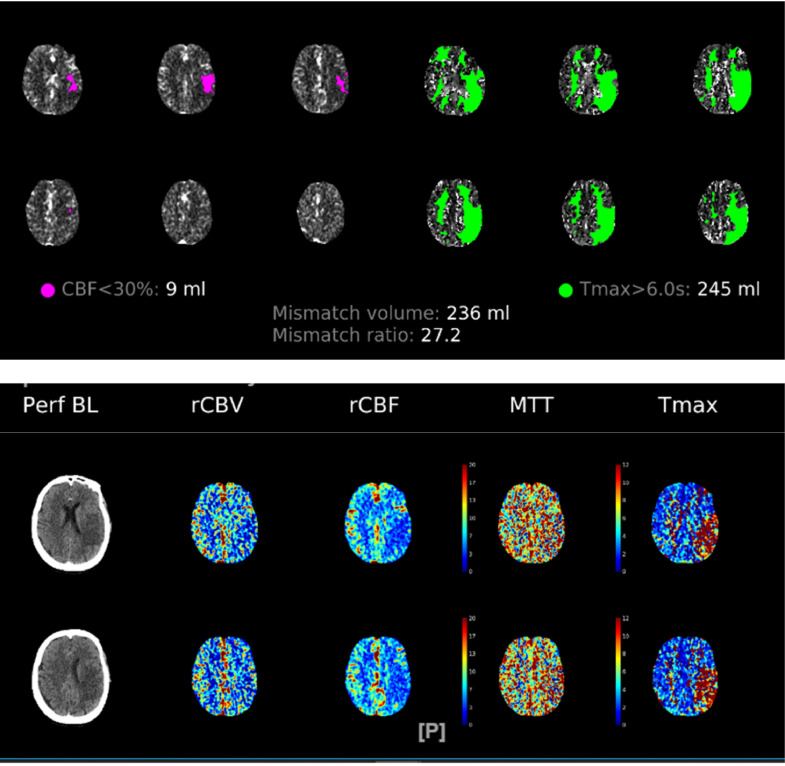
Automated brain computed tomography perfusion imaging, hypoperfusion area is seen in the infarcted region in the left frontoparietotemporal region (CBF<30% [9] in corresponding to 9 mL ischemic core, and Tmax > 6s accounts for hypoperfusion area of 245 mL, with mismatch volume of 236 mL and ratio of 27.2).
